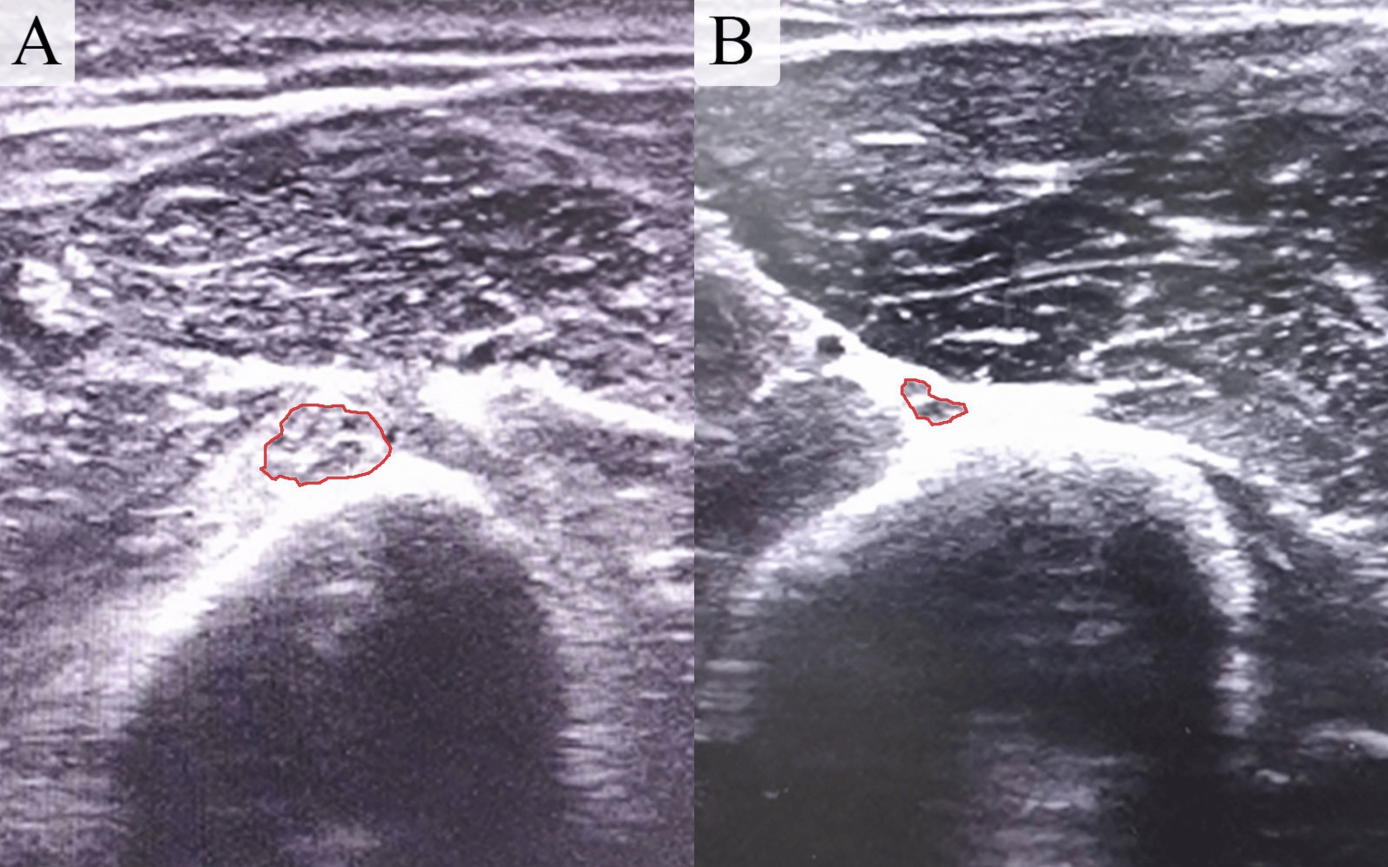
Enlarged images obtained through comparative ultrasound scans of the left and right arms reveal a damaged radial nerve with a cross-sectional area (CSA) of 0.22 cm² in the left arm (image A), in contrast to a normal radial nerve with a CSA of 0.05 cm² in the right arm (image B).
